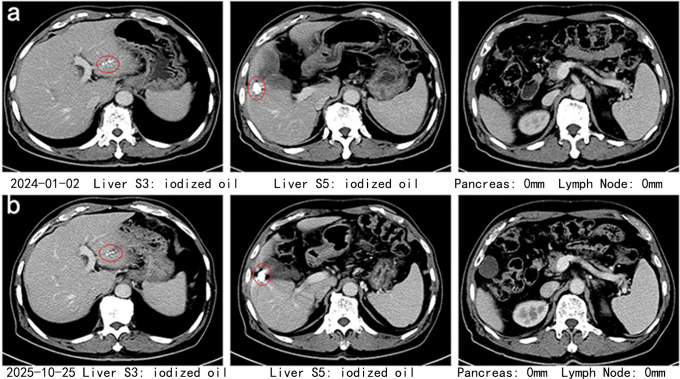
Long-term follow-up imaging confirming sustained complete response. (a) CT scan (January 2024) and (b) CT scan (October 2025) demonstrated a sustained complete response (CR), with complete resolution of the previously seen pancreatic primary lesion and metastatic lymph nodes. Only stable, non-enhancing iodized oil deposits remain in the liver, confirming the absence of viable tumor (Table 1).
